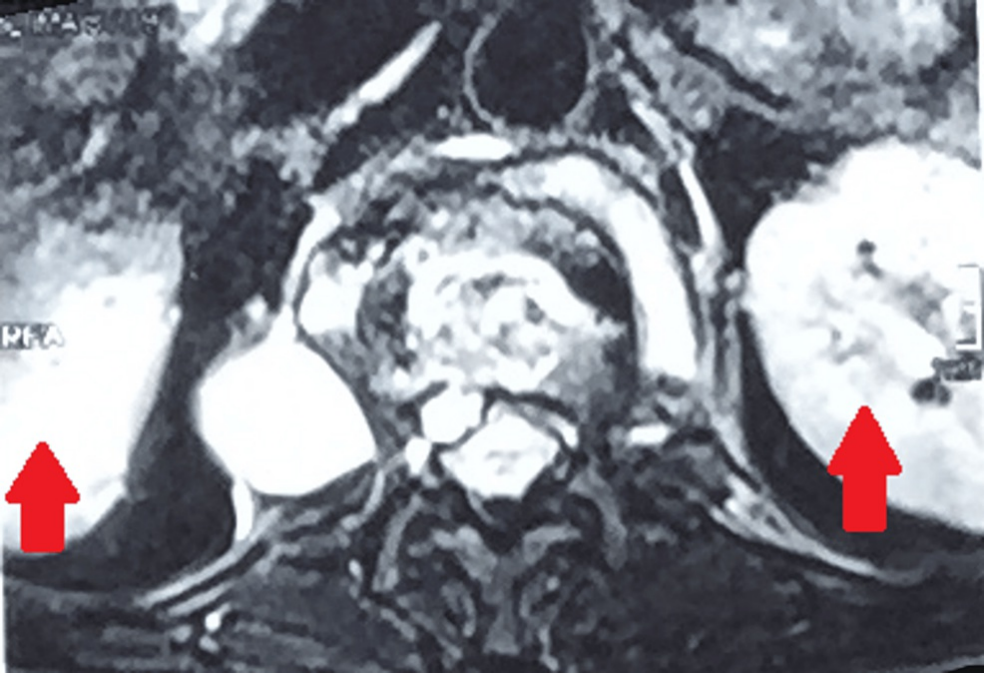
MRI of the dorso-lumbar spine showing bilateral psoas abscesses
